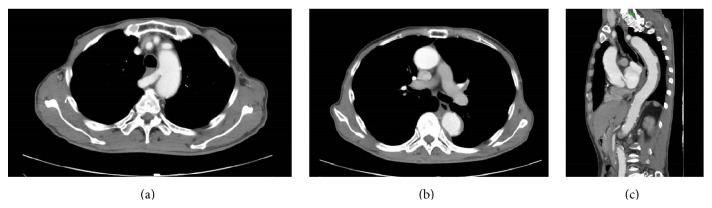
Contrast-enhanced CT findings after onset. (a) Horizontal cross section. False lumen thrombosis was observed. No changes in the origin of the right subclavian artery were noted. (b) Horizontal cross section. (c) Coronal cross section. The emergence of ULP in the descending aorta was observed.
